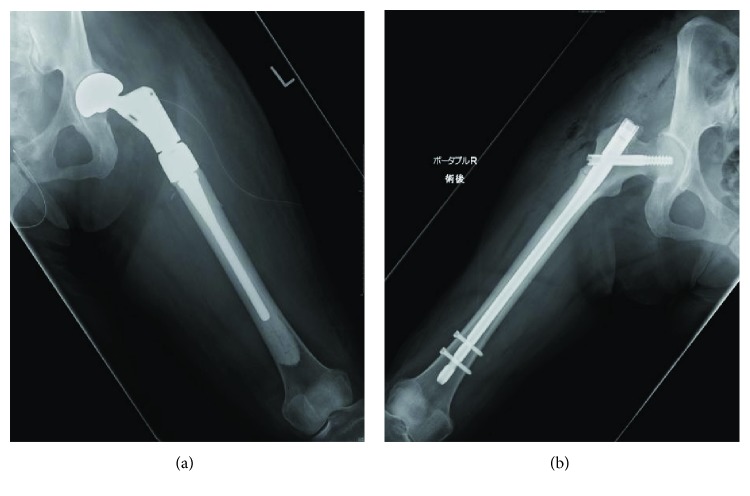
Postoperative images of bilateral atypical femoral fractures (AFFs). (a) Radiography after endoprosthetic reconstruction for the left displaced AFF. (b) Radiography after prophylactic intramedullary nailing for the right incomplete AFF.
